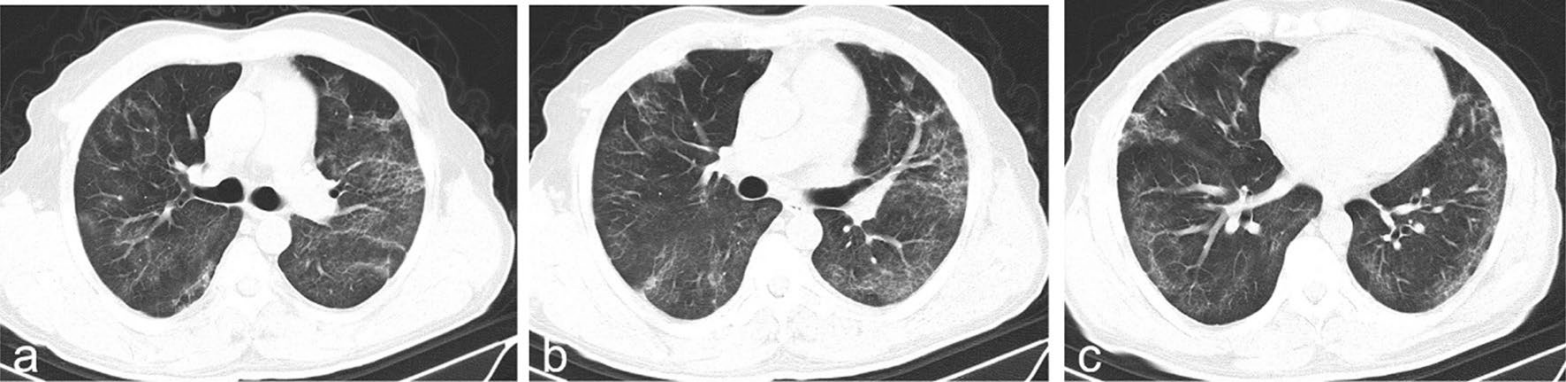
Transverse CT scans in a man with infected with SARS-CoV-2. Scan obtained on 20th day from onset of symptoms shows bilateral, light ground glass opacities with irregular linear opacities. The patient was discharged from hospital 11 days after the scan.
